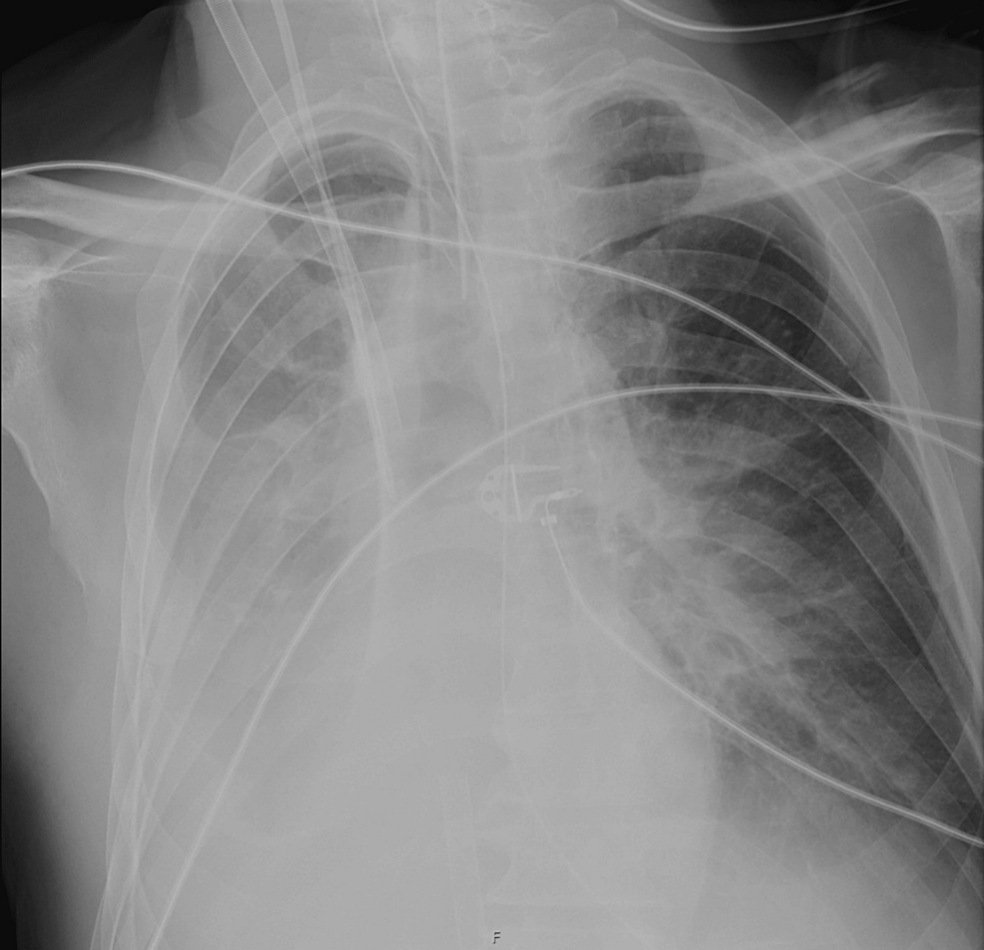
The patient's X-ray in the ICU ICU: Intensive care unit
